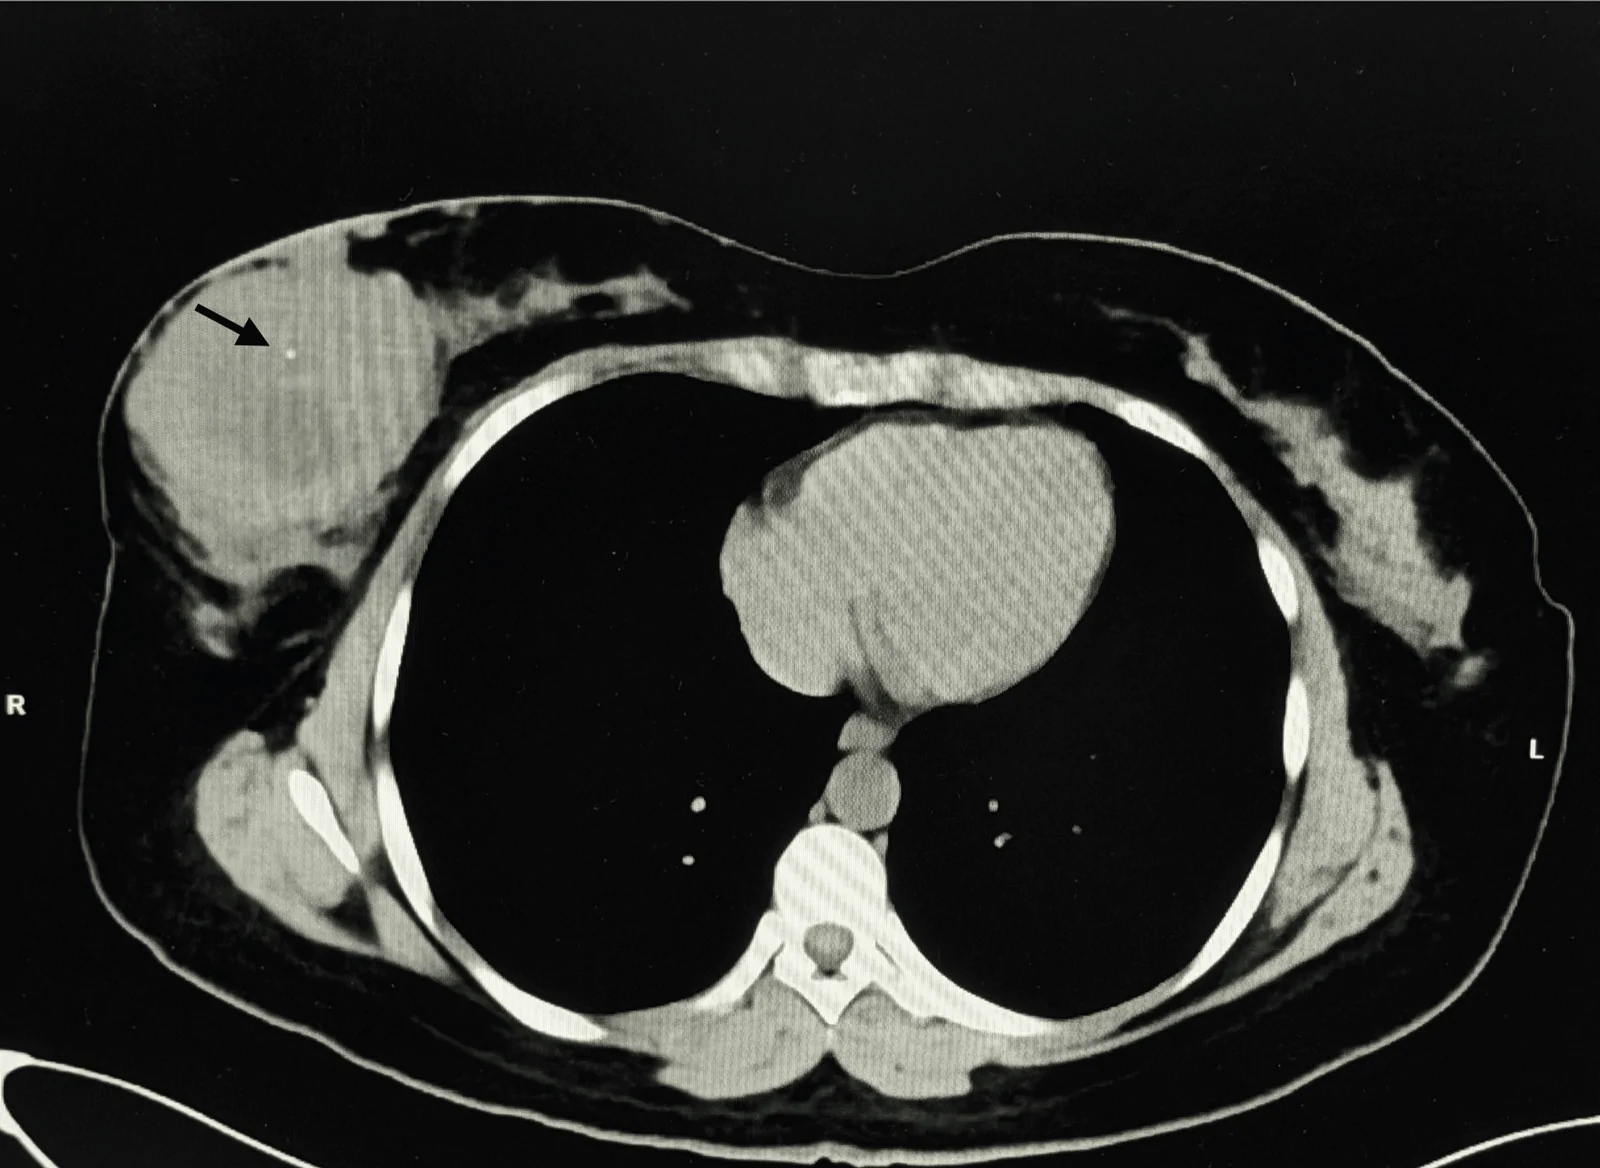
Staging CT (chest cut): Index mass in the right breast, with no evidence of lung metastasis. A core biopsy marker clip seen in the right breast (black arrow).
